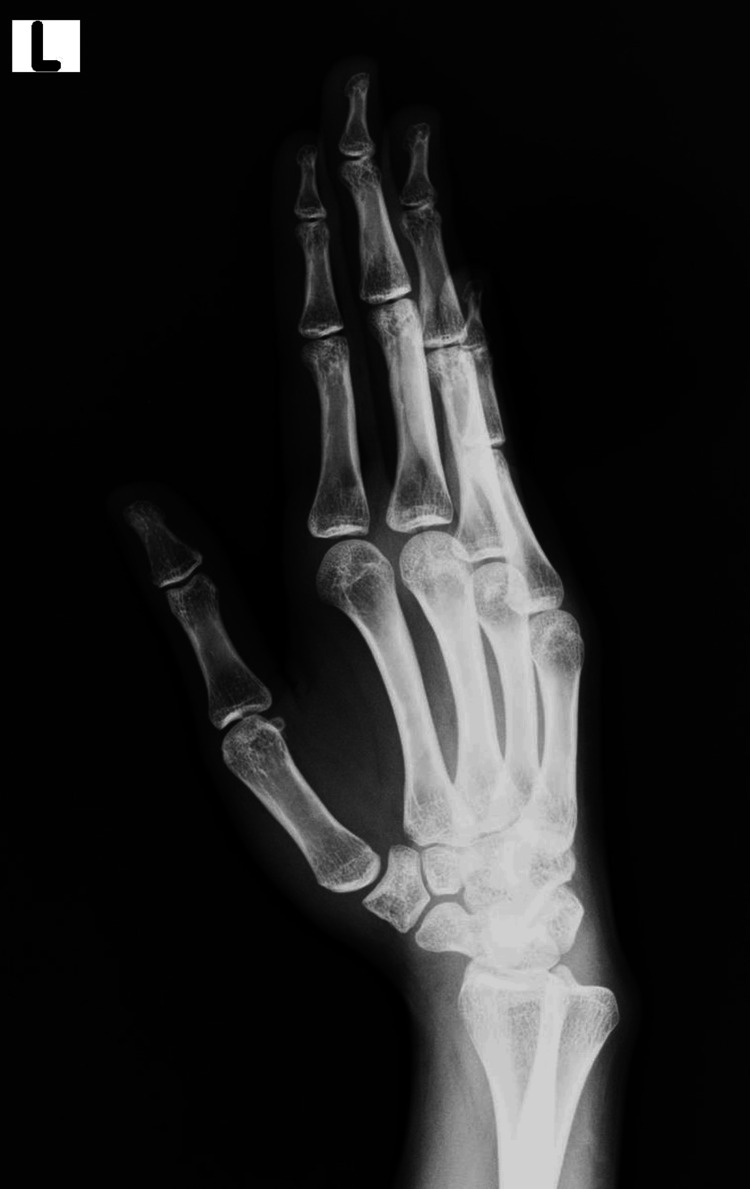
Radiographic results of the true lateral view of the thumb obtained by pronating the hand 20° and then angling the beam 15° to 20° distal.
